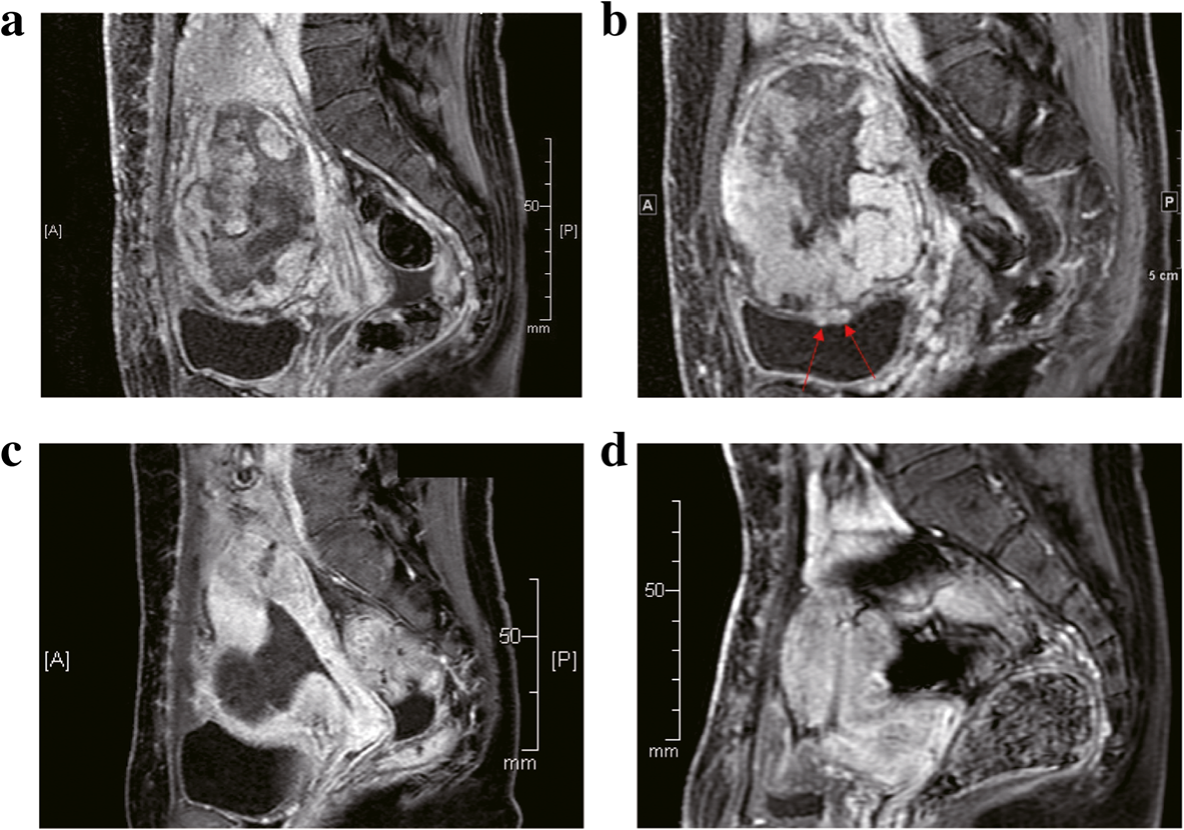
A pelvic MRI was performed on postoperative days 48 (a and b), 92 (c), and 232 (d). a and b, MRI on postoperative day 48 revealed a decrease in the size of the placenta (approximately 12 cm). Gadolinium-enhanced T1-weighted MRI revealed a diffuse lesion with variable enhancement of the placenta. The other slice of the image is shown in b. Red arrows in (b), the residual placenta that had invaded the bladder wall. c, MRI on postoperative day 92 revealed a further decrease in the size of the placenta (approximately 6 cm). Gadolinium-enhanced T1-weighted MRI exhibited no enhancement of the placenta. d, MRI on postoperative day 232 confirmed the absence of any residual placenta. With gadolinium enhancement, a small defect was observed in the uterine anterior wall
